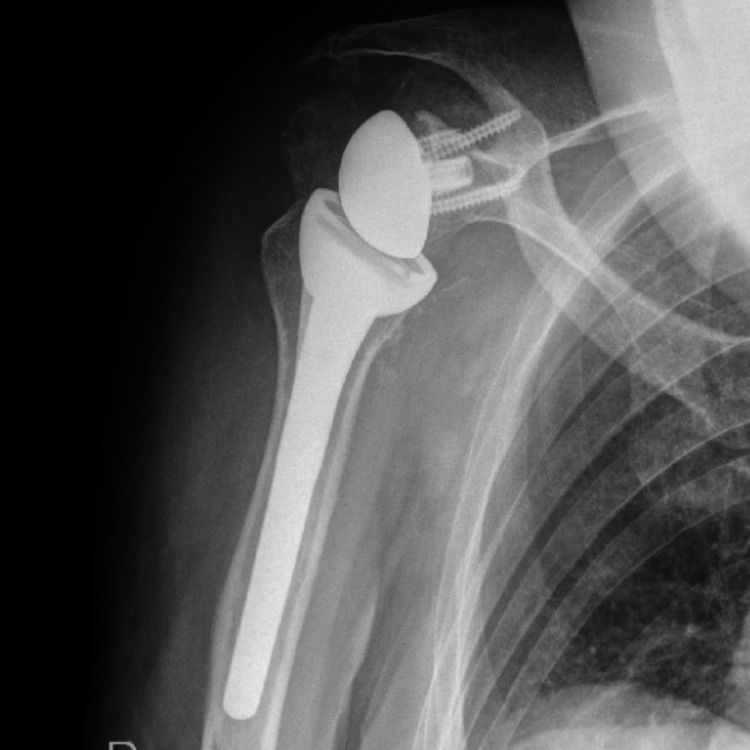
Postoperative shoulder radiograph The component of the right reverse total shoulder arthroplasty was in a satisfactory position.
